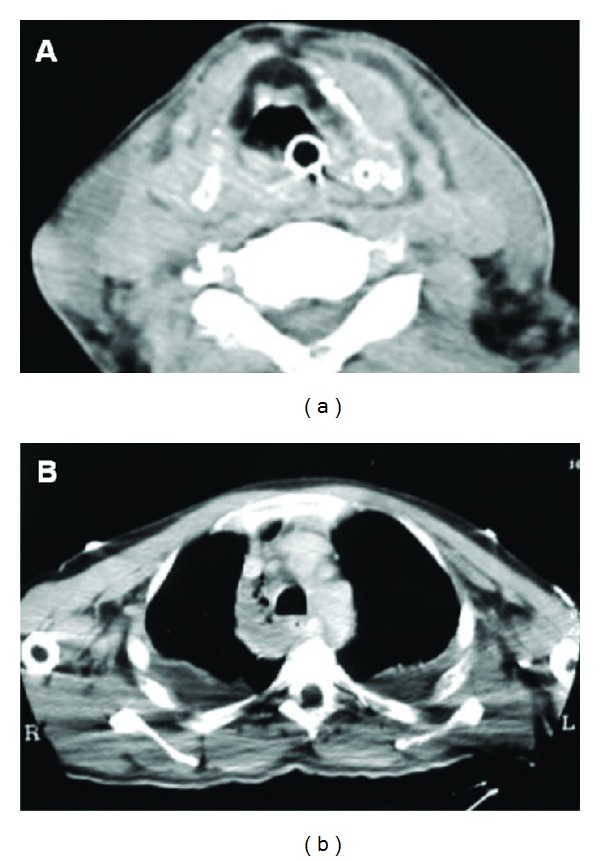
Patient number 1—CT images. (a) Area of effusion of liquid mixed with gas is visible between muscle planes in the cervical area. (b) Expansion of mediastinum due to presence of liquid and gas can be easily observed and a pleural effusion is clearly visible bilaterally.
